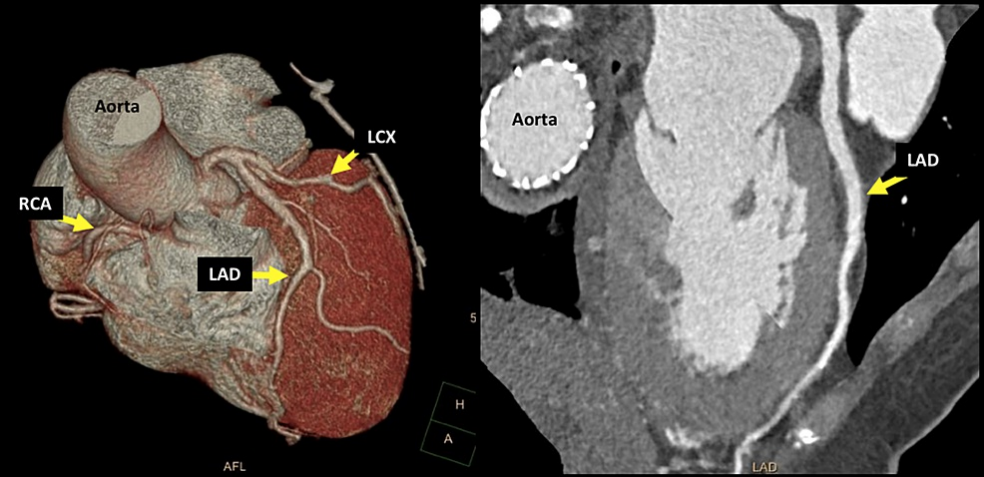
CTA showing ordinary course and caliber of LAD artery fully opacified without luminal narrowing or stenosis or evidence of dissection. CTA, computed tomography angiography; LAD, left anterior descending; LCX, left circumflex artery; RCA, right coronary artery
